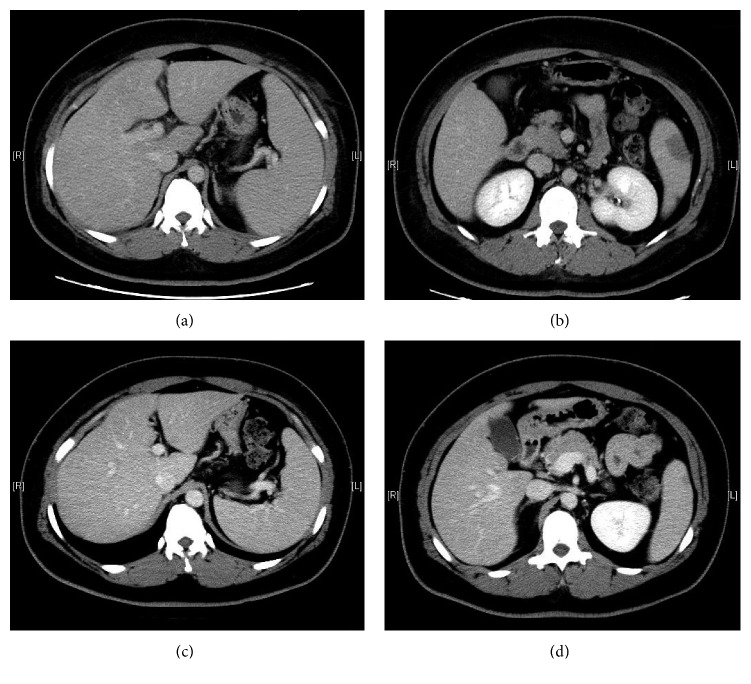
Abdominal computed tomography at admission. (a) Enlarged liver and spleen are shown. (b) A geographical low attenuation area is observed on the dorsal side of the spleen, which suggests splenic infarction. These findings were improved after ganciclovir treatment. (c) Enlarged liver and splenomegaly were improved. (d) The geographic low attenuation area in the spleen was decreased.
